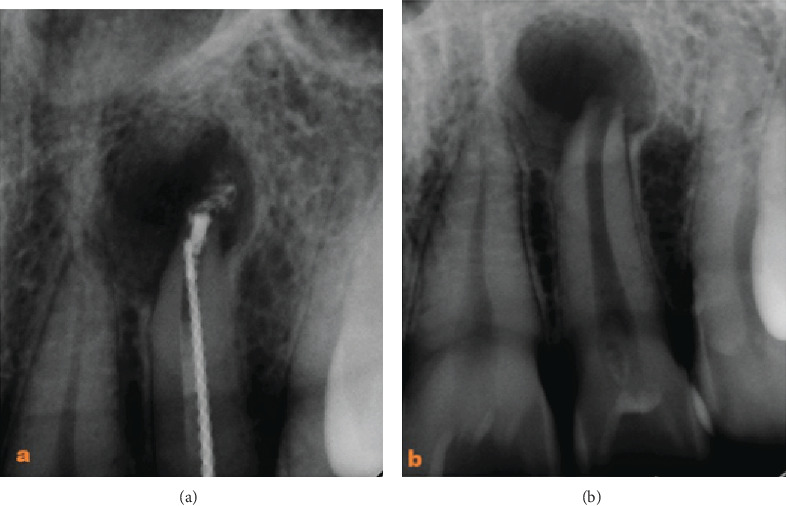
Periapical radiograph of tooth 22 after GP removal with a Hedstrom file.
